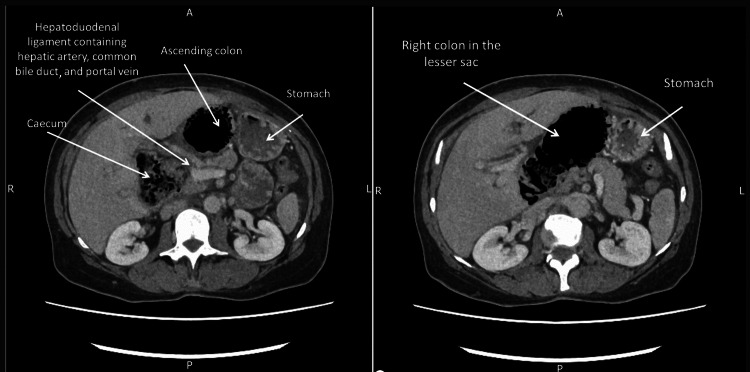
Axial CT scan images showing the right colon in the lesser sac with pneumatosis intestinalis suggesting ischaemia (right) and demonstrating the herniated content behind the hepatoduodenal ligament (left).
